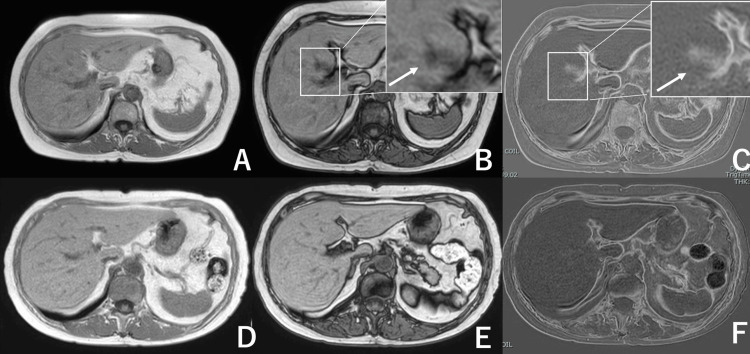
Magnetic resonance imaging (MRI) demonstrates intralesional fat and subsequent complete resolution A: T1-weighted in-phase image shows a focal lesion in segment IV (S4); B: the lesion shows marked signal loss on the opposed-phase image compared with the in-phase image, consistent with intralesional fat (arrow); C: diffusion-weighted imaging shows no diffusion restriction (arrow) (magnified insets are shown in the upper right corners of panels B and C); D–F: follow-up MRI three months after intensive glycemic control shows complete disappearance of the lesion on in-phase and opposed-phase images and no abnormality on diffusion-weighted imaging. DWI: diffusion-weighted imaging; MRI: magnetic resonance imaging; S4: segment IV
